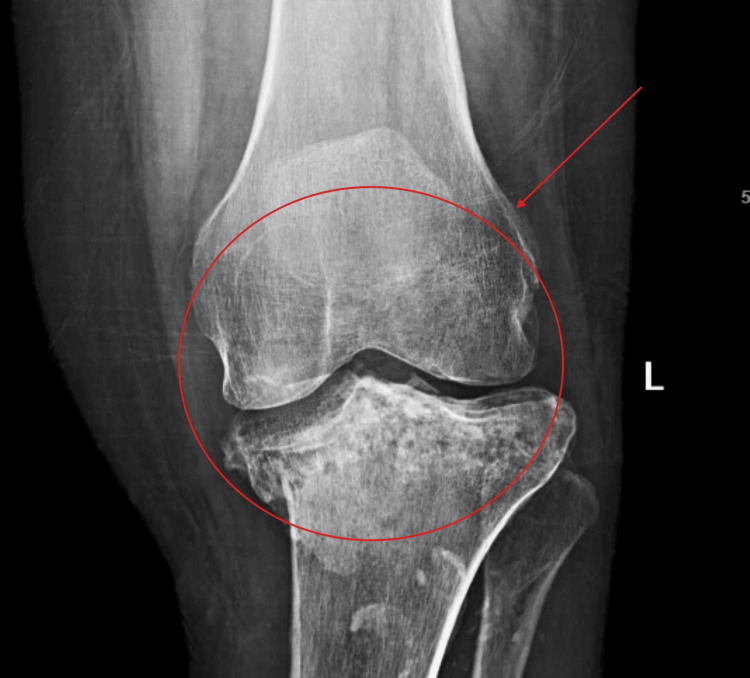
Baseline knee X-ray showing a proximal tibial lesion (2019)
